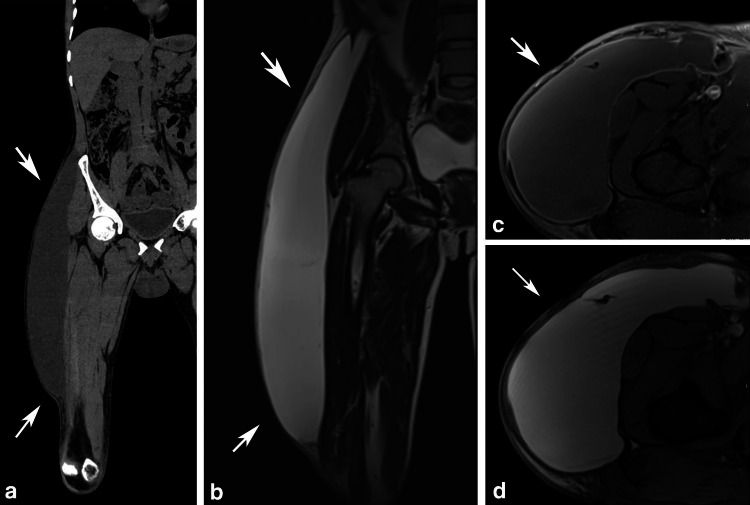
Cross-sectional imaging using non-contrast CT (a) & MRI (b and c) confirmed the characteristic subcutaneous location and extent of the lesion. The collection demonstrated intermediate signal on T2-weighted images (b and d) and intermediate signal with a thin partial capsular enhancement in the T1-weighted post-contrast image (c) (a) Coronal non-contrast CT image of the thigh and hip, (b) Coronal T2-weighted MRI of the thigh and hip, (c) Axial post-contrast fast-suppressed T1-weighted MRI of the thigh, and (d) Axial T2-weighted MRI of the thigh White arrows indicate the extent of the collection. CT: Computed tomography, MRI: Magnetic resonance imaging
